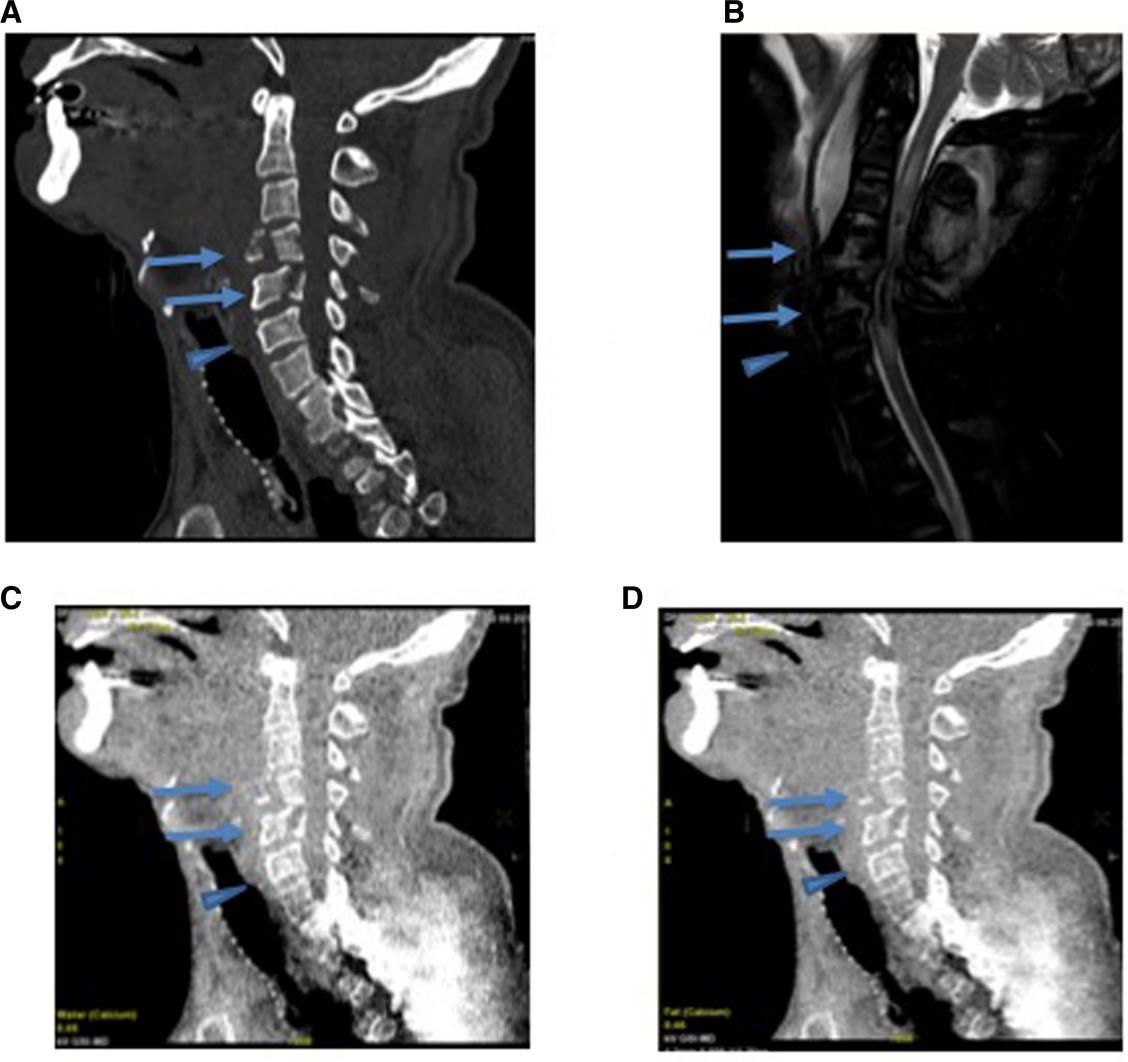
A 57-year-old male presenting with an acute cervical fracture after a 50-ft fall based on GSI dual-energy CT analysis of with MRI correlate. (A) The conventional CT scan of the cervical spine demonstrates fractures of C4 and C5 vertebral bodies (arrow) and adjacent uninvolved C6 vertebral body (arrowhead). (B) The STIR imaging of the cervical spine demonstrates an increased signal in the region of the fractures (arrows) without signal abnormality in the uninvolved C6 (arrowhead). (C) Water (calcium) and (D) fat (calcium) images show no significant change in water content between the fractured C4 and C5 (arrow) and normal C6 (arrowhead).
